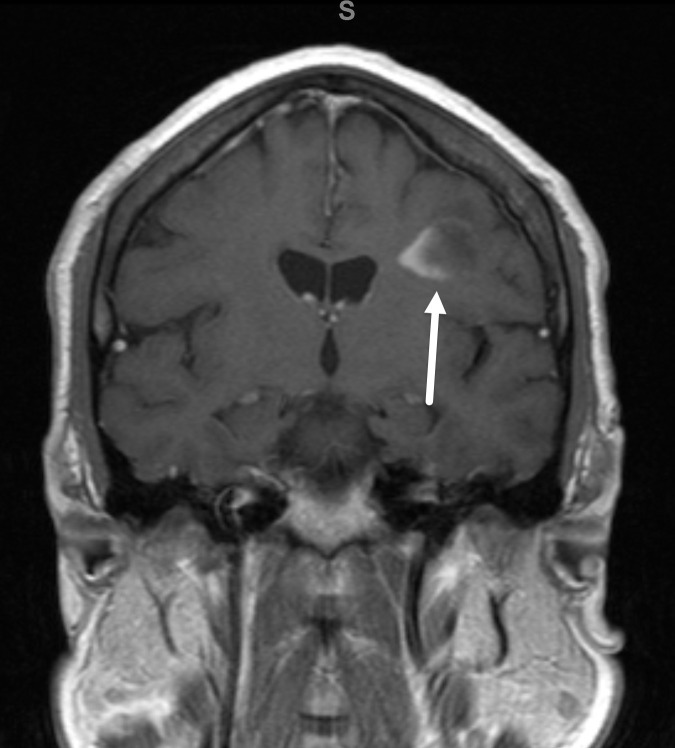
Incomplete rim enhancement of the left frontal lobe lesion. Post-contrast coronal T1 MR image through the lesion in Figure 2 demonstrates incomplete rim enhancement open to the cortical surface (arrow). This finding is typical of demyelination.
